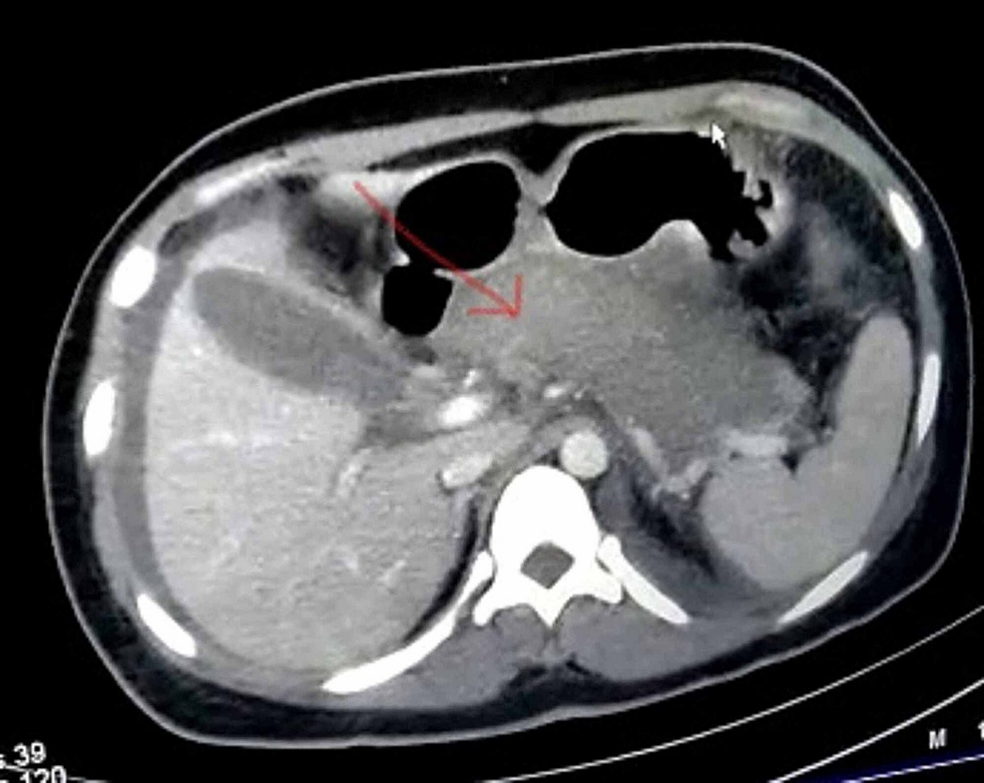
Contrast-enhanced computed tomographic scan showing diffuse enlargement of pancreas with hypo-enhancement (red arrow)
